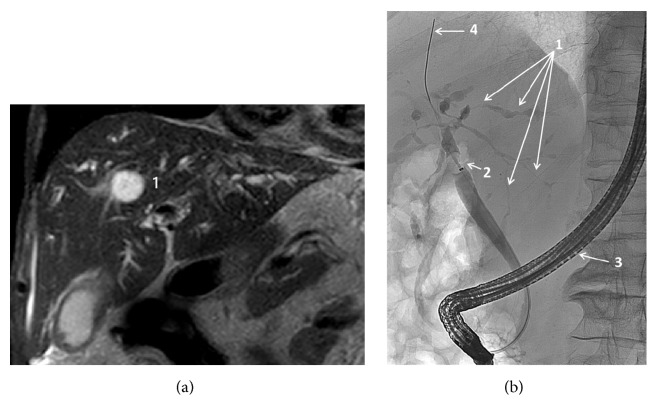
Imaging findings in a patient with IgG4-related sclerosing cholangitis (IgG4-SC). (a) At magnetic resonance imaging, no tumor-like lesions are seen. A benign hepatic cyst is indicated (1). (b) Diffuse and multiple intrahepatic biliary stenoses and dilatations at endoscopic retrograde cholangiography (1). The stenoses are relatively long. In classical primary sclerosing cholangitis (PSC), the stenoses would have been shorter, giving the cholangiogram a more beaded appearance. No complete obstruction of a bile duct is seen, a finding that would have been more characteristic of bile duct carcinoma. 2: occlusive balloon, 3: endoscope, 4: guide wire.
